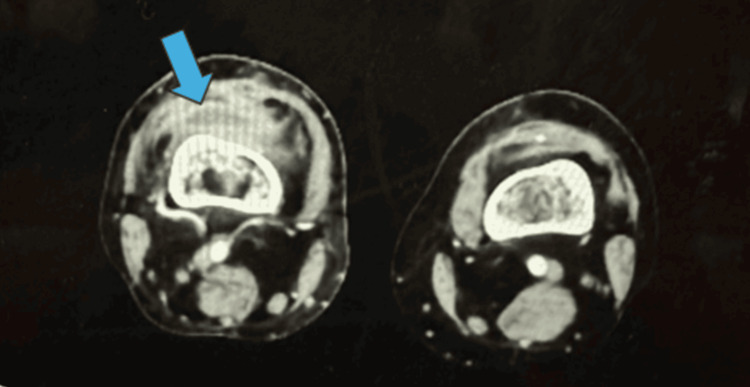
Case 4 CT angiography CT angiography of both knees showing effusion in the right knee, as indicated by the arrow.
